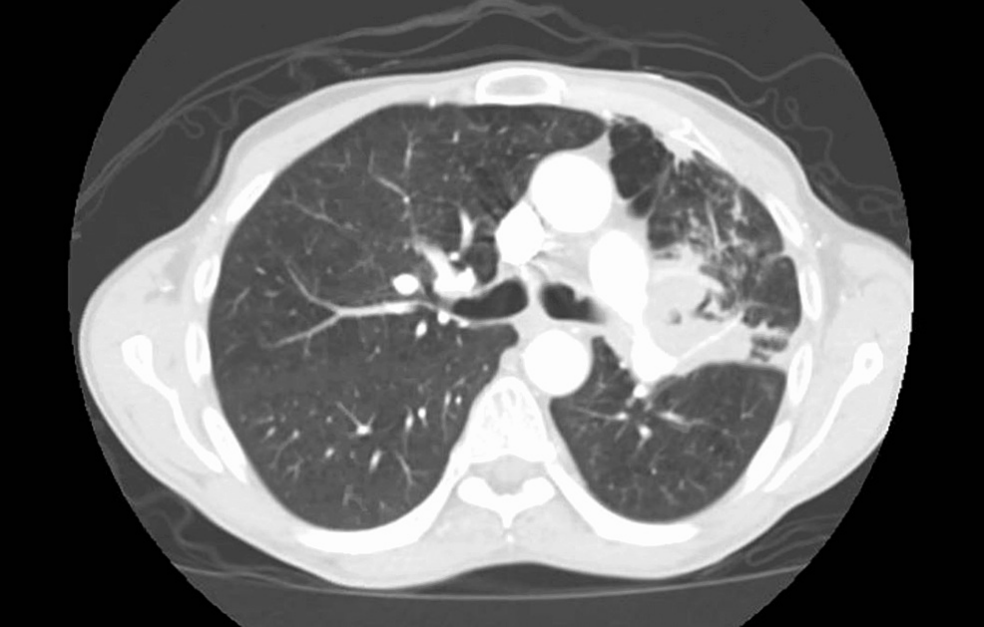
CT of the chest showing 2.7 X 1.6 X 1.3 cm cavitary lesion in the left lower lobe
